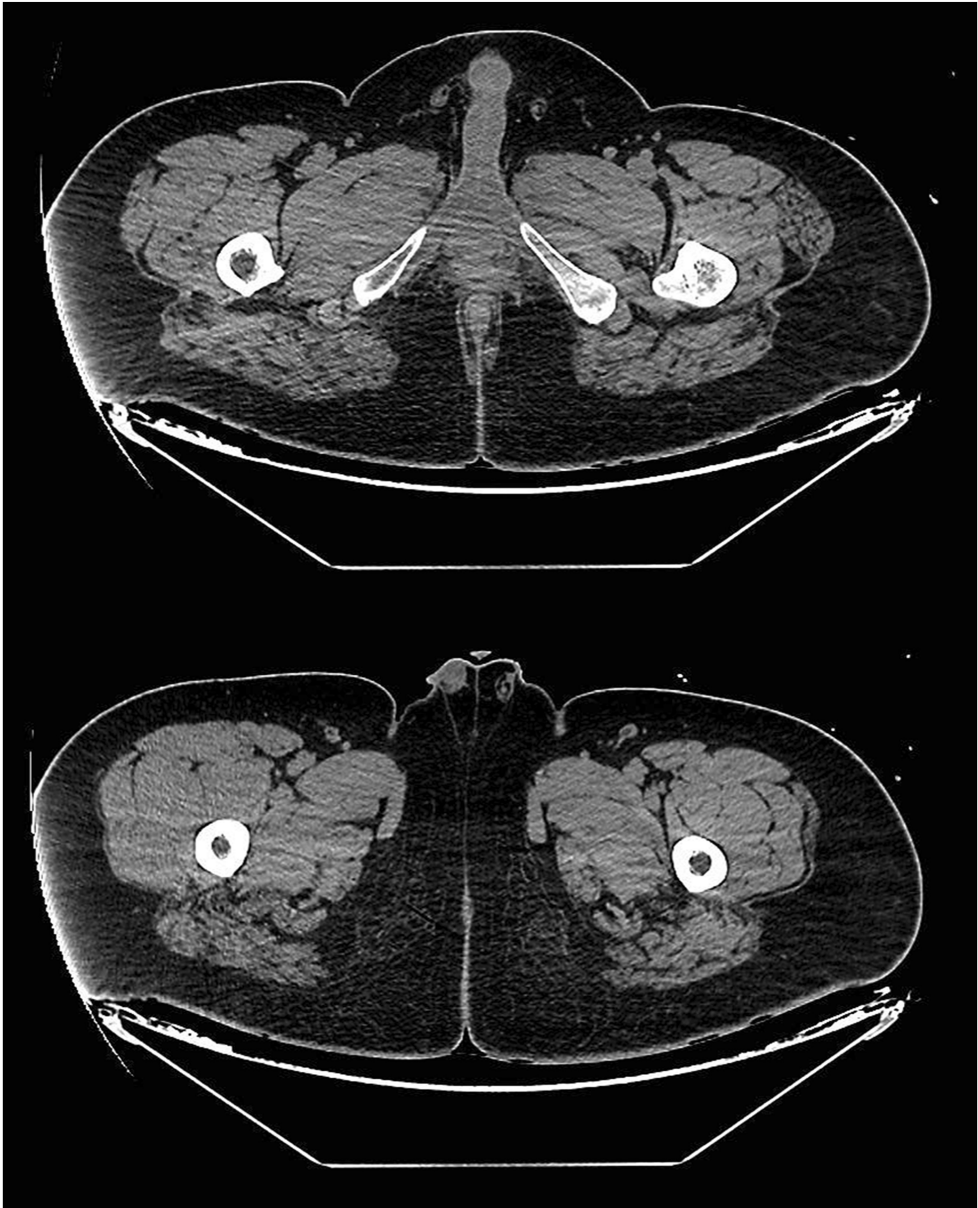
CT scan revealing a small penis and small testicles.
